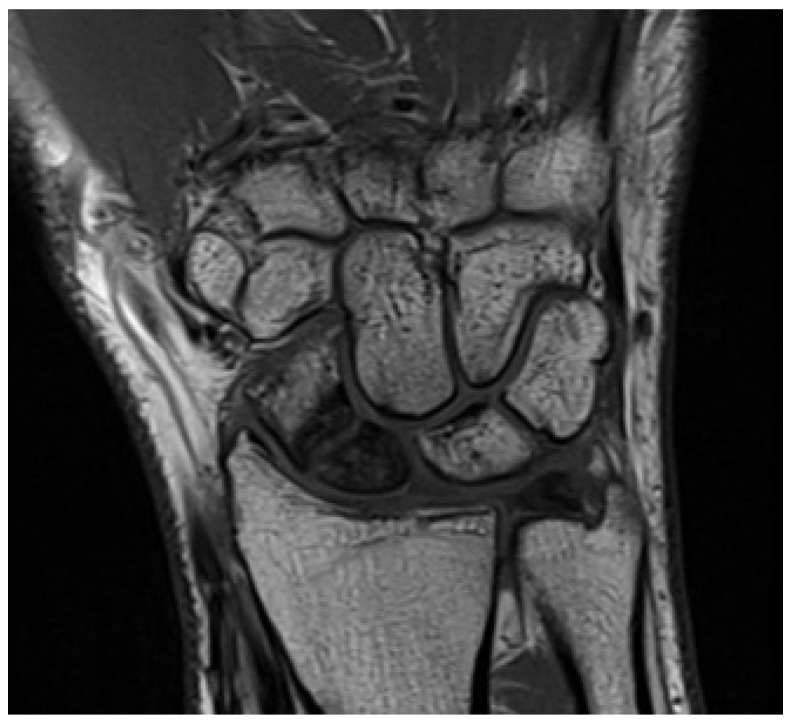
Wrist MRI evaluation of the soft tissues (intact TFCC) following a scaphoid nonunion fracture.
